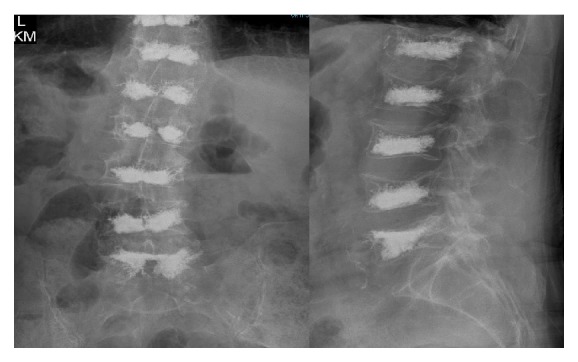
AP and lateral X-rays of the lumbar spine following the last kyphoplasty. The patient underwent one kyphoplasty of each lumbar vertebral body.
